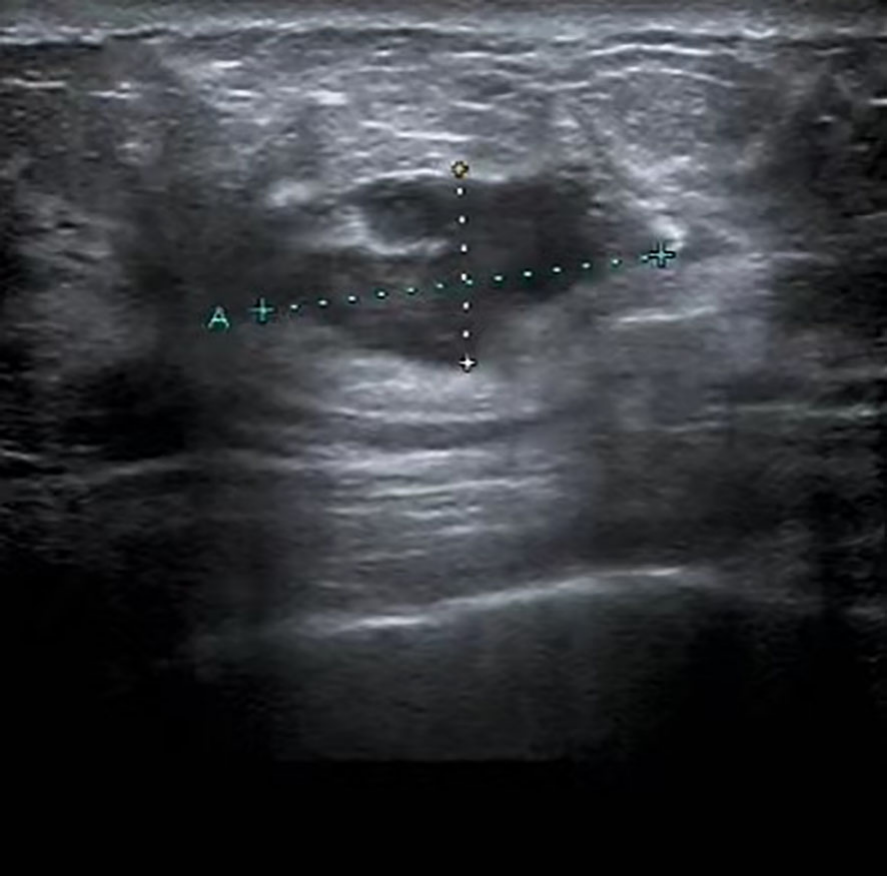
Ultrasound image of the breast: A mass, measuring 25.4 mm by 12.5 mm, was identified in the upper quadrant of the right breast, near the three o’clock position. This mass has indistinct margins and an irregular contour.
